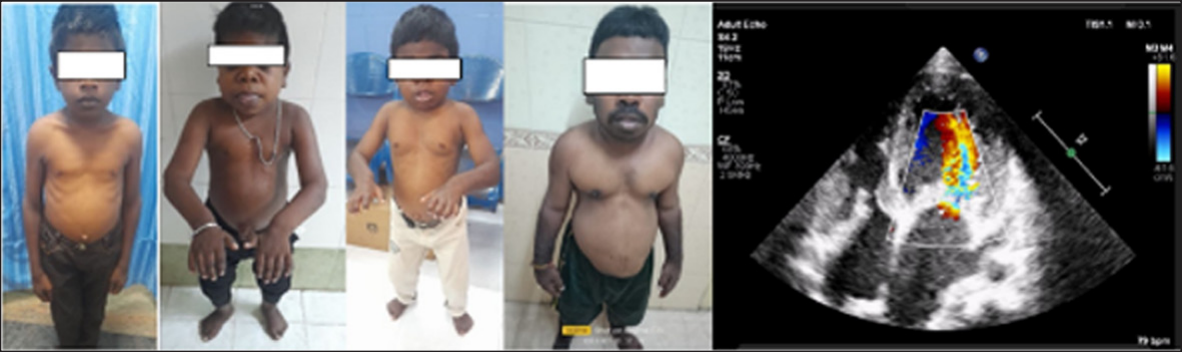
Photos of patients previously diagnosed with mucopolysaccharidosis with and an echocardiographic assessment.
